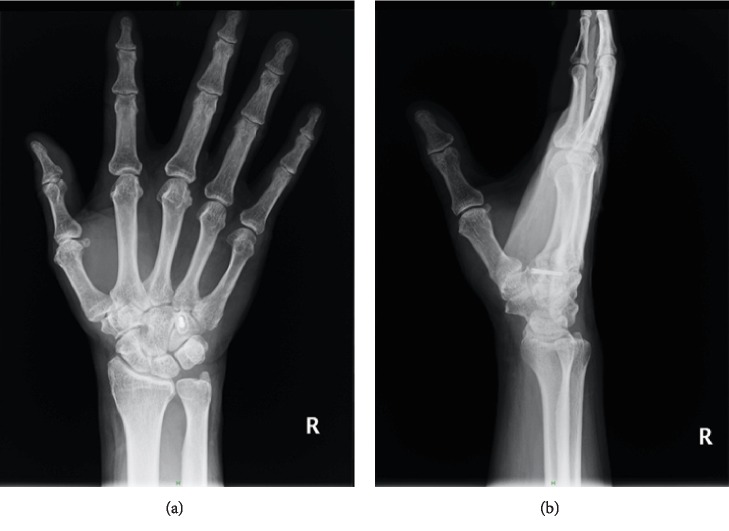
Radiograph 2 years after the surgery. (a) Posteroanterior view. (b) Oblique semisupinated view.
